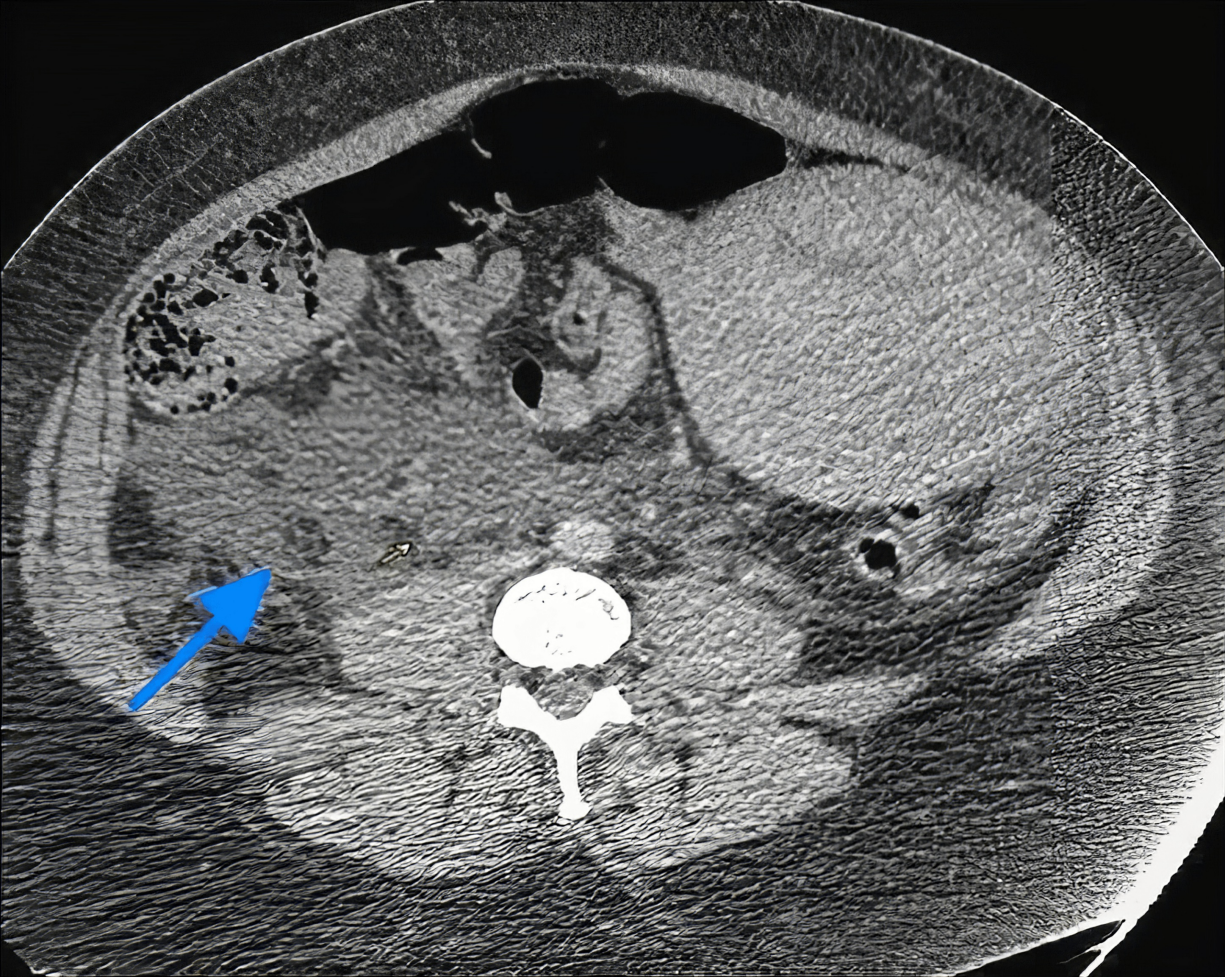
CT post-contrast portal venous phase axial image showing moderate free-fluid collection with air foci adjacent to the lower segment of the right ureter (arrow). An enlarged post-partum uterus can be noted on the left side of the lower abdomen.
